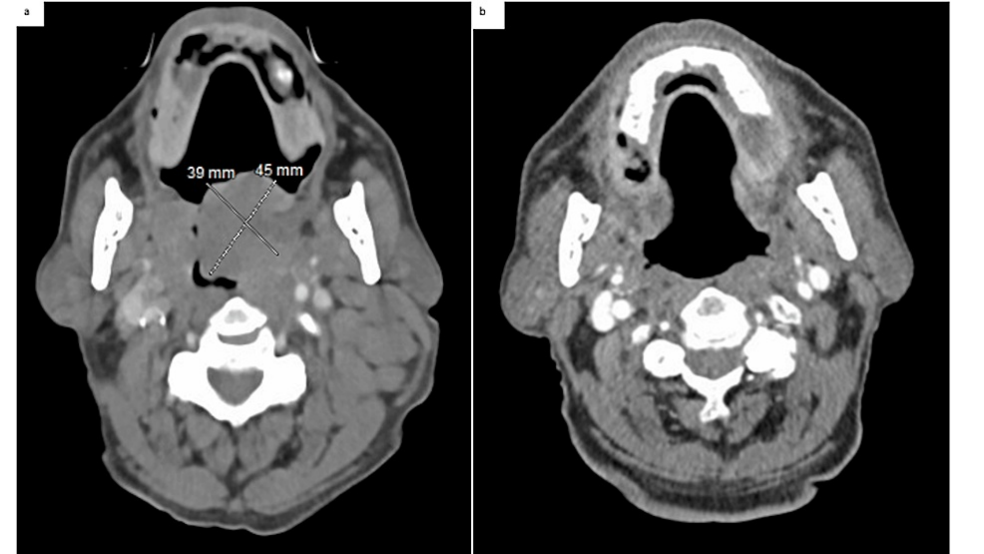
Pre- and post-treatment computed tomography (a) Enlargement of bilateral palatine tonsils, left greater than right. Left measures 4.5 x 3.9 cm. (b) Imaging post-treatment with radiation and systemic therapy (rituximab, bendamustine, and ibrutinib) showed resolution of previously reported enlargement of the palatine tonsils and bilateral cervical lymphadenopathy.
